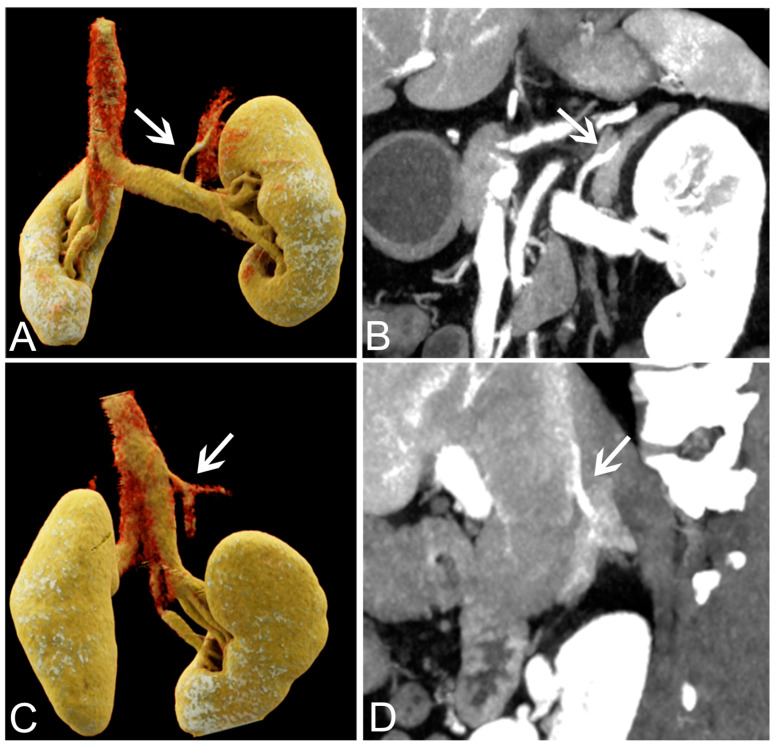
VR and MIP reconstructions of the adrenal veins in dual-energy computed tomography images. VR (A) and MIP (B) images of the left adrenal vein (white arrows) in a 42-year-old female patient with a left adrenal adenoma. VR (C) and MIP (D) images of the right adrenal vein (white arrows) in a 42-year-old female patient with a right adrenal adenoma. VR, volume-rendered; MIP, maximum-intensity projection.
